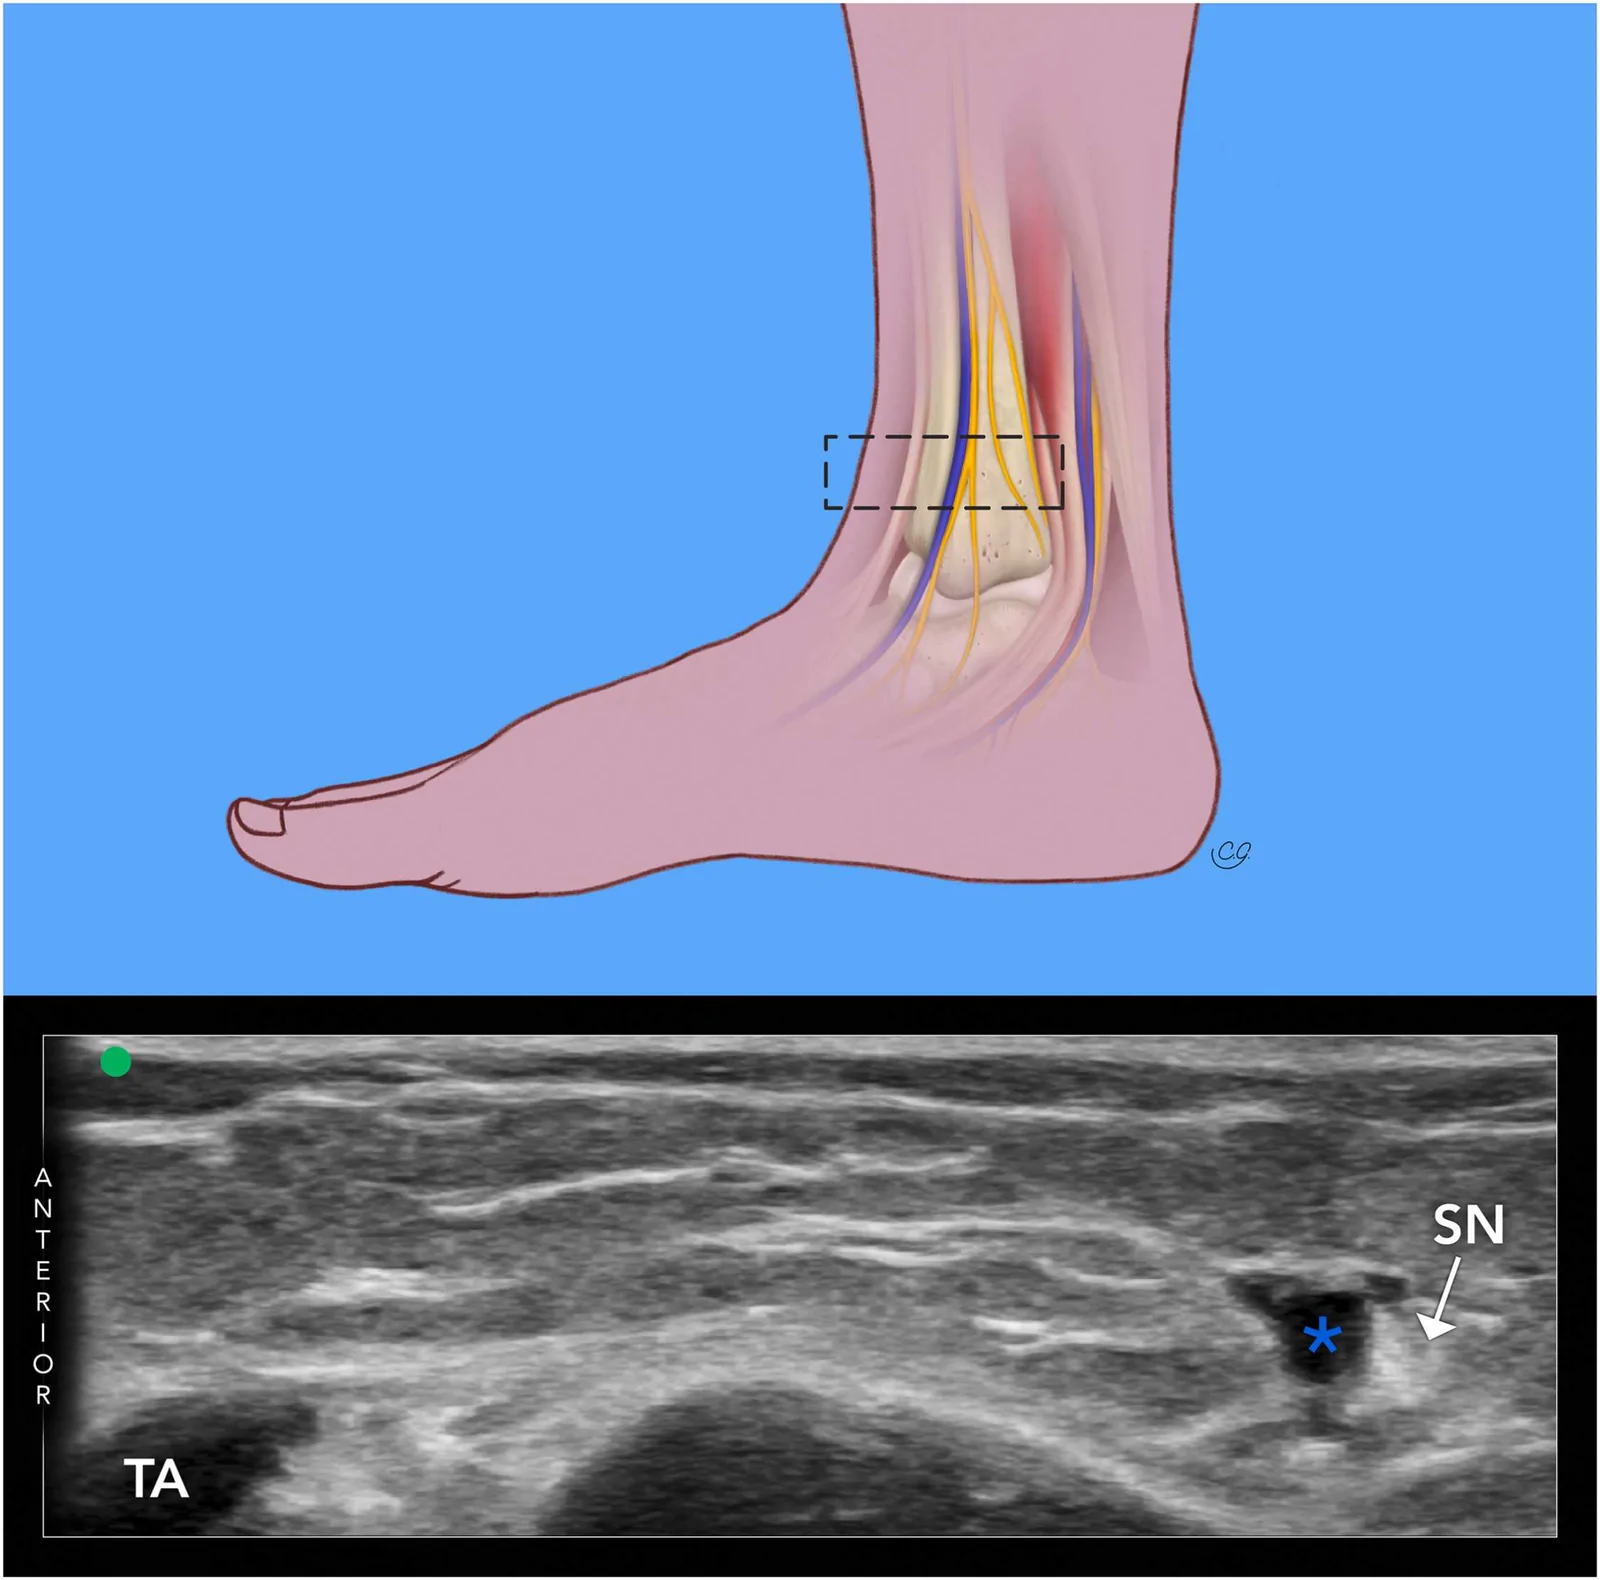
Ultrasound image and probe position for identification of the saphenous nerve. Please note that the anterior branches of the saphenous nerve can be visualized in the subcutaneous plane between the tibialis anterior tendon and the midpoint of the medial malleolus. Only the posterior branches are missed, but they do not innervate the forefoot and midfoot. *: saphenous vein, SN: saphenous nerve, TA: tibialis anterior tendon. Reproduced with permission from Girón Arango Medicine Professional Corporation.
